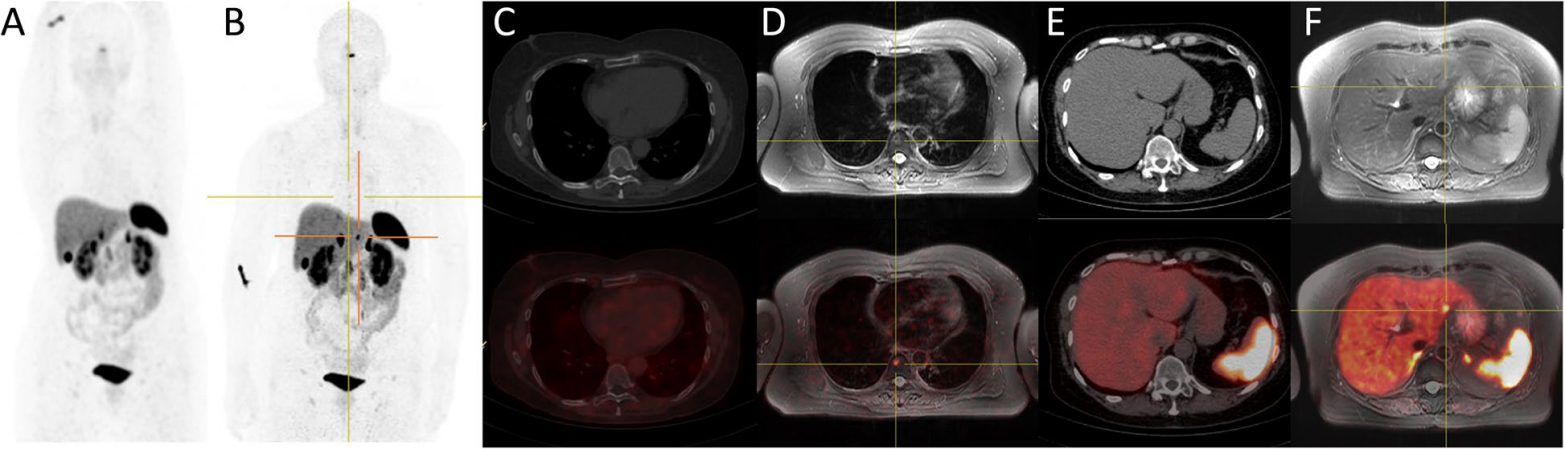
Comparative images between PET/CT and PET/MRI in patient number 15. PET/MRI revealed a new bone lesion (involvement of a new organ) and a new hepatic lesion (organ already affected). A Maximum intensity projection (MIP) in PET/CT. B MIP in PET/MRI. C PET/CT in bone reconstruction. D PET/MRI in FS T2-weighted sequence. A new lesion is detected in the T8 soma showing a central signal hypointensity and a peripheric hyperintensity signal, with intense uptake on PET. E PET/CT in soft tissue reconstruction. F PET/MRI in FS T2-weighted sequence. A new hepatic lesion is observed in segment II, hyperintense in FS T2- weighted sequence and with intense uptake in PET. This uptake was difficult to discriminate on PET/CT from hepatic baseline heterogeneous activity
